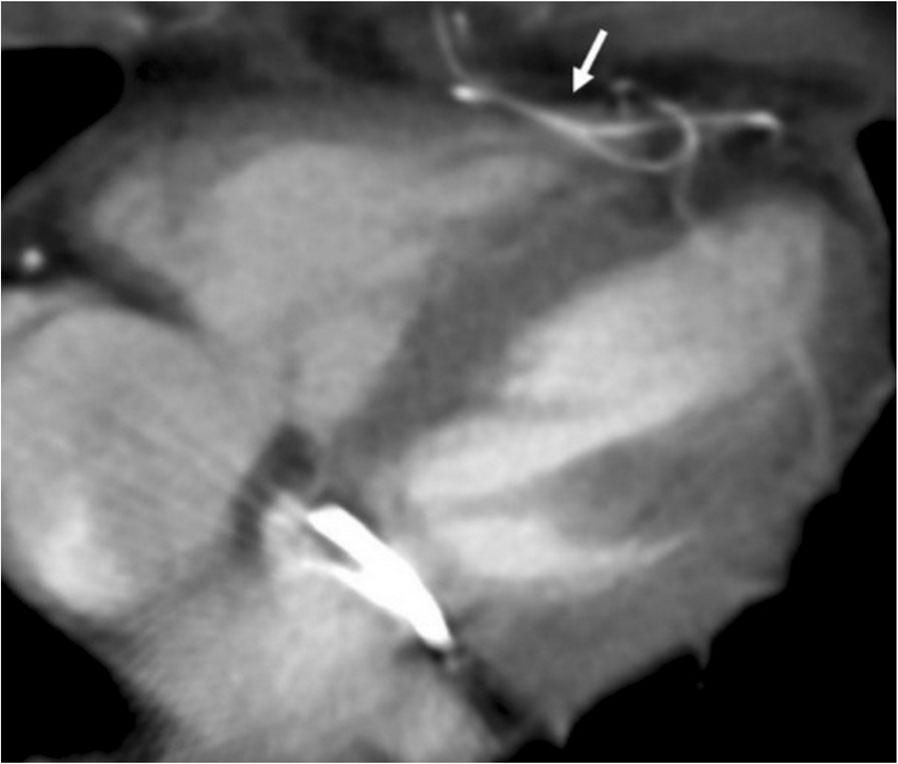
A 45-year-old woman recently underwent mitral valve replacement surgery. Axial contrast-enhanced CT image reveals temporary epicardial pacemaker wires (arrow)
